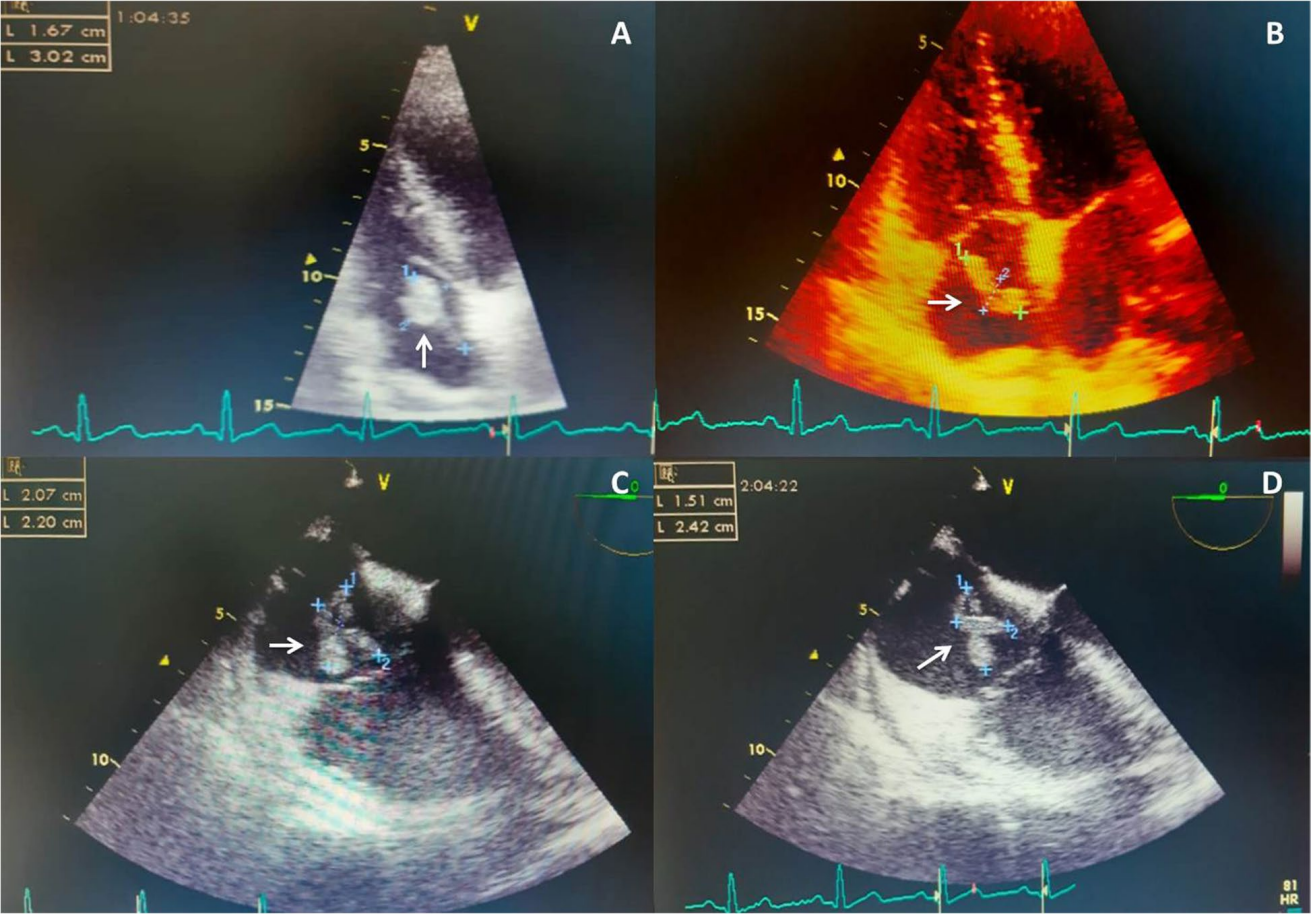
A, B Trans-thoracic echocardiography. C, D Trans-esophageal echocardiography. The imaging assessments showed a large lobulated mass (Clot) in right atrium attached to Chiari network (Arrow)
